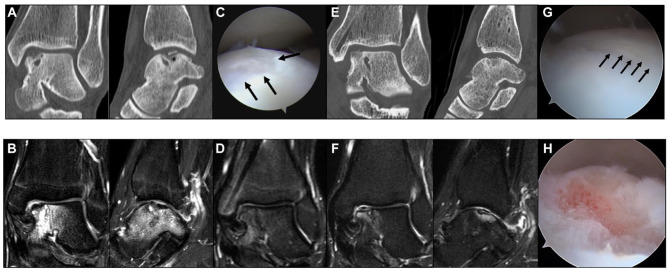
Case 2. (A) Pre-operative CT images. (B) Pre-operative MRI. (C) Arthroscopic findings. Arrows indicate fibrous tissues. (D) Post-operative MRI at 1 year after surgery. (E) Post-operative CT at 6 years after surgery. (F) Post-operative MRI at 6 years after surgery. (G) Arthroscopic findings. Arrows indicate a fissure between the osteochondral fragment and surrounding normal cartilage. (H) After resection of the osteochondral fragment.
